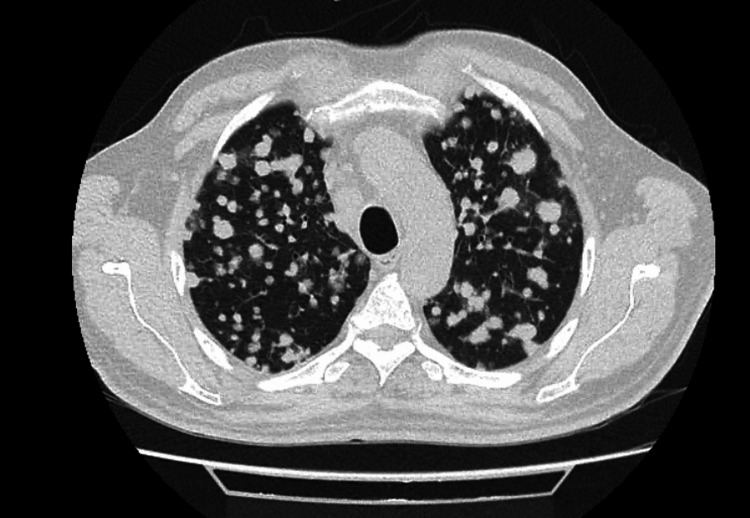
Chest CT scan revealing massive pulmonary metastases (Case 2) Extensive pulmonary metastases displaying a “balloon-release” pattern.
